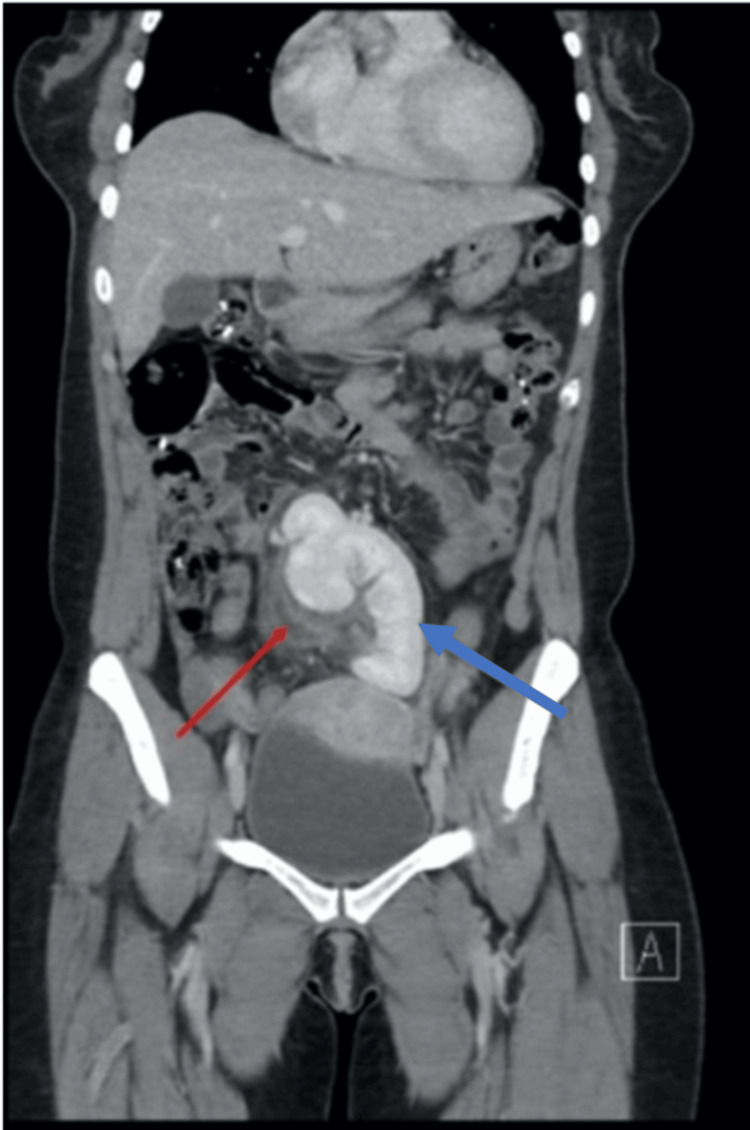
Coronal contrast-enhanced computed tomography (CT) of the abdomen demonstrates malrotation and ectopia of the left kidney, which is visualized in the midline of the lower abdomen (blue arrow). A filling defect within the left renal vein (red arrow) is seen, consistent with renal vein thrombosis.
